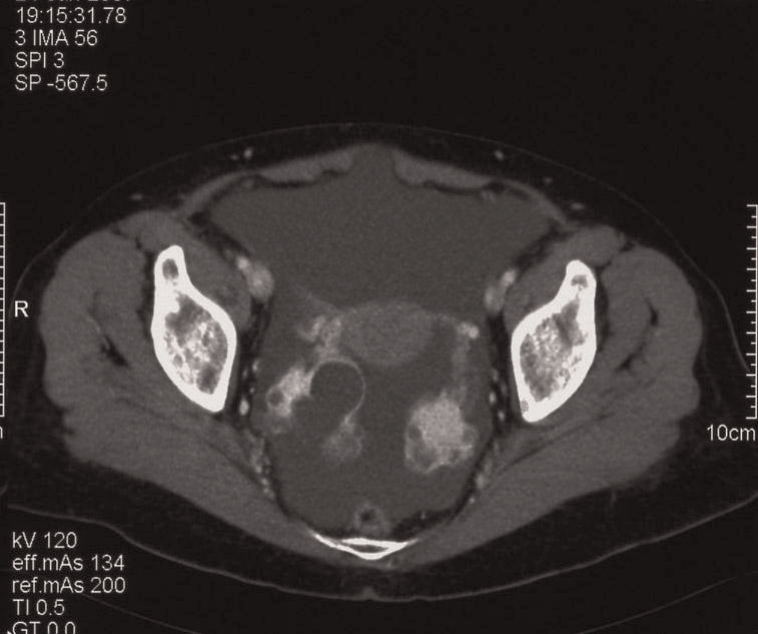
Contrast enhanced CT, portal phase. Presence of bilateral mostly solid parenchymatous ovarian masses with poor contrast enhancement and regular and smooth margins. The scan shows no uterine abnormalities.
